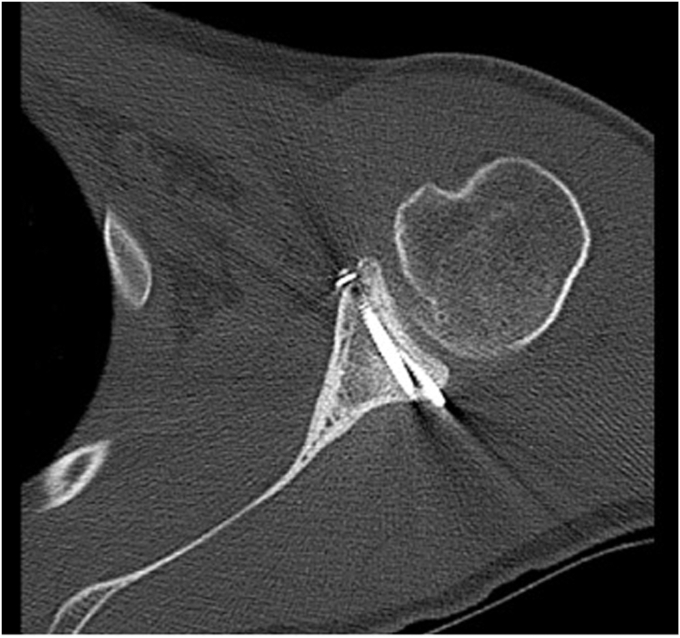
Axial CT image taken after posterior dislocation revealing reverse Hill-Sachs lesion.
